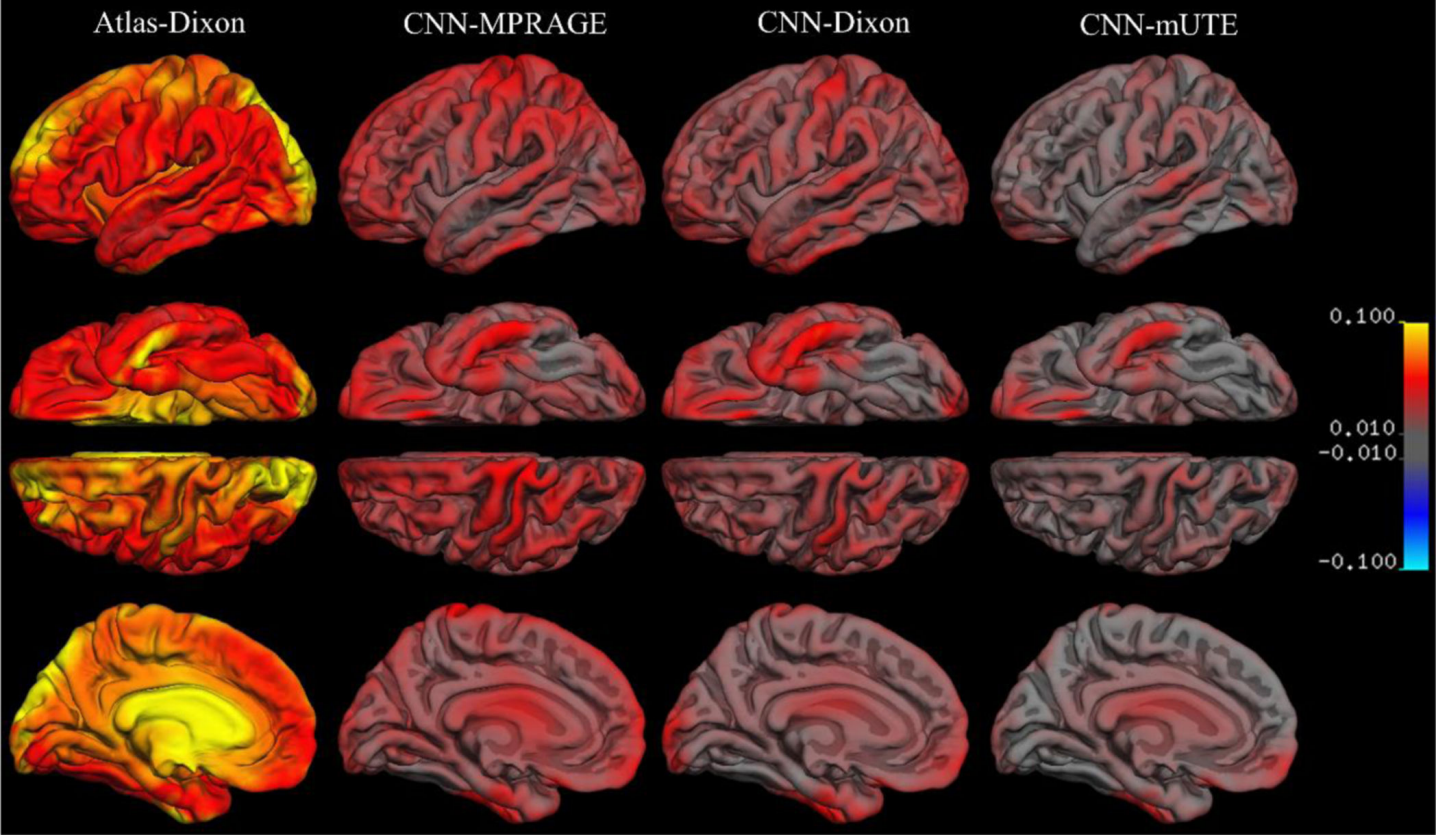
The averaged surface maps of SUVR relative error for different methods: Atlas-Dixon (first column), CNN-MPRAGE (second column), CNN-Dixon (third column) and CNN-mUTE (fourth column). Only the right hemisphere of the surface map is shown. The color map range is from 1% to 10% in magnitude.
